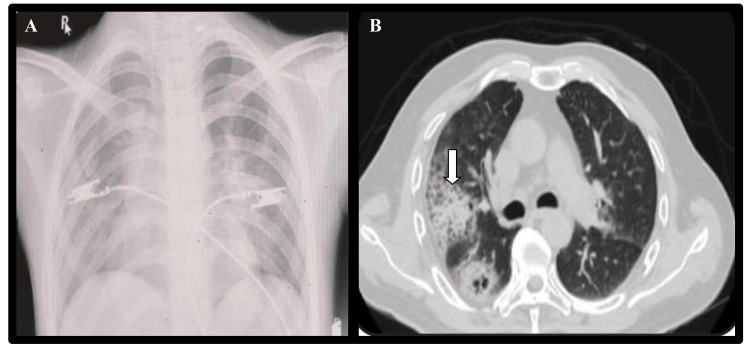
(A) Chest radiograph showing multiple bronchoalveolar opacities with diffuse haziness (black arrow) (B) High-resolution computed tomography (HRCT) chest showing necrotizing/cavitating pneumonia (white arrow)
